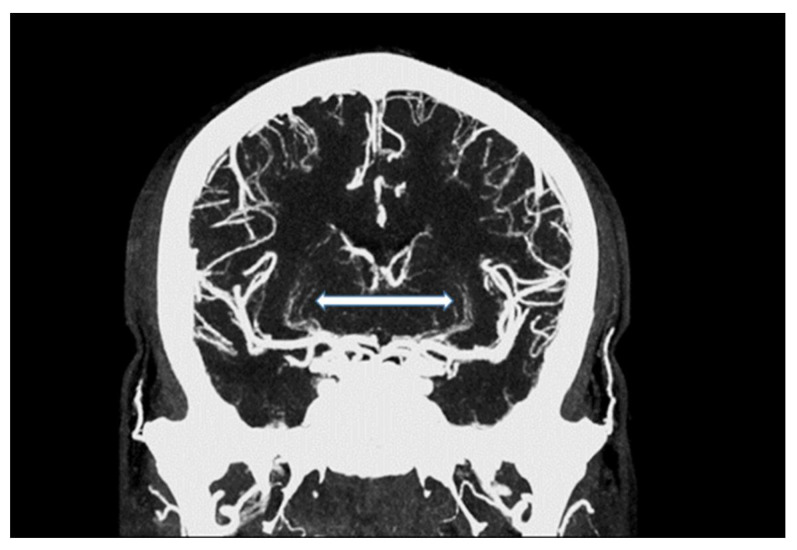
Maximum intensity projection of an intracranial UHR-CTA, depicting traceability of lenticulostriate arteries (LSA; white arrow).
